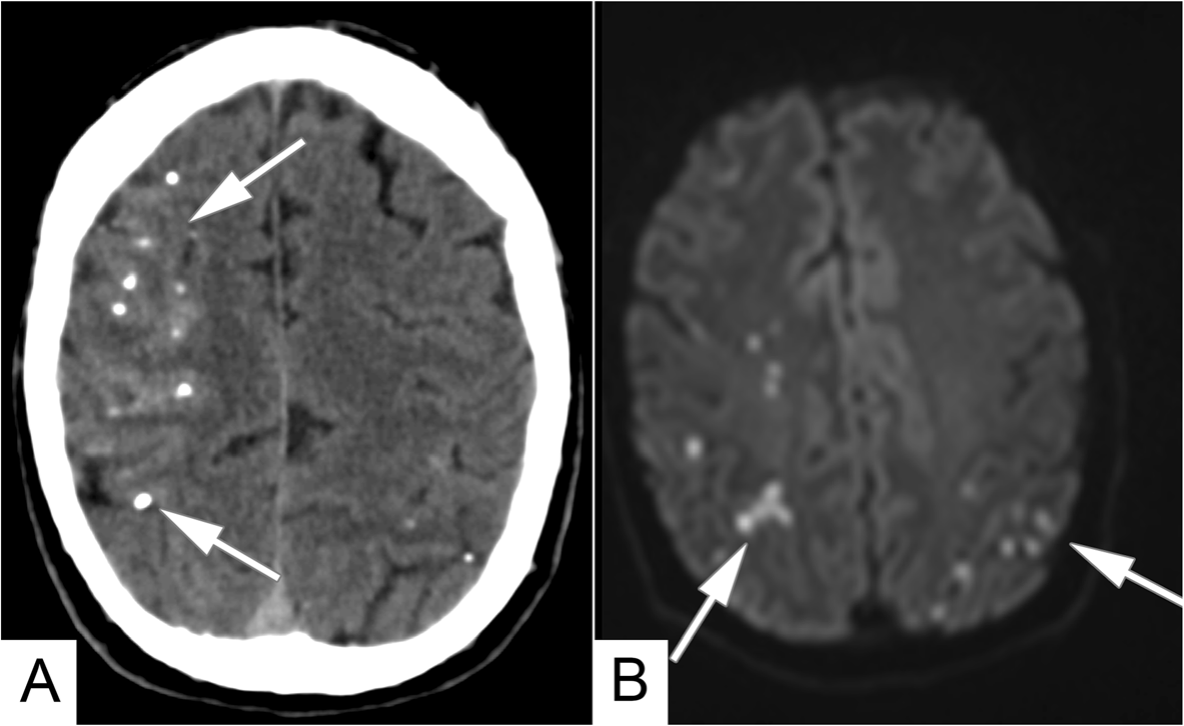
(a) Post-procedural non-enhanced axial CT. Multiple glue deposits in the right frontal and parietal lobes (white arrows). (b) Same day axial DWI MRI (b = 1000). of the brain showing acute infarcts both in right and left hemispheres
